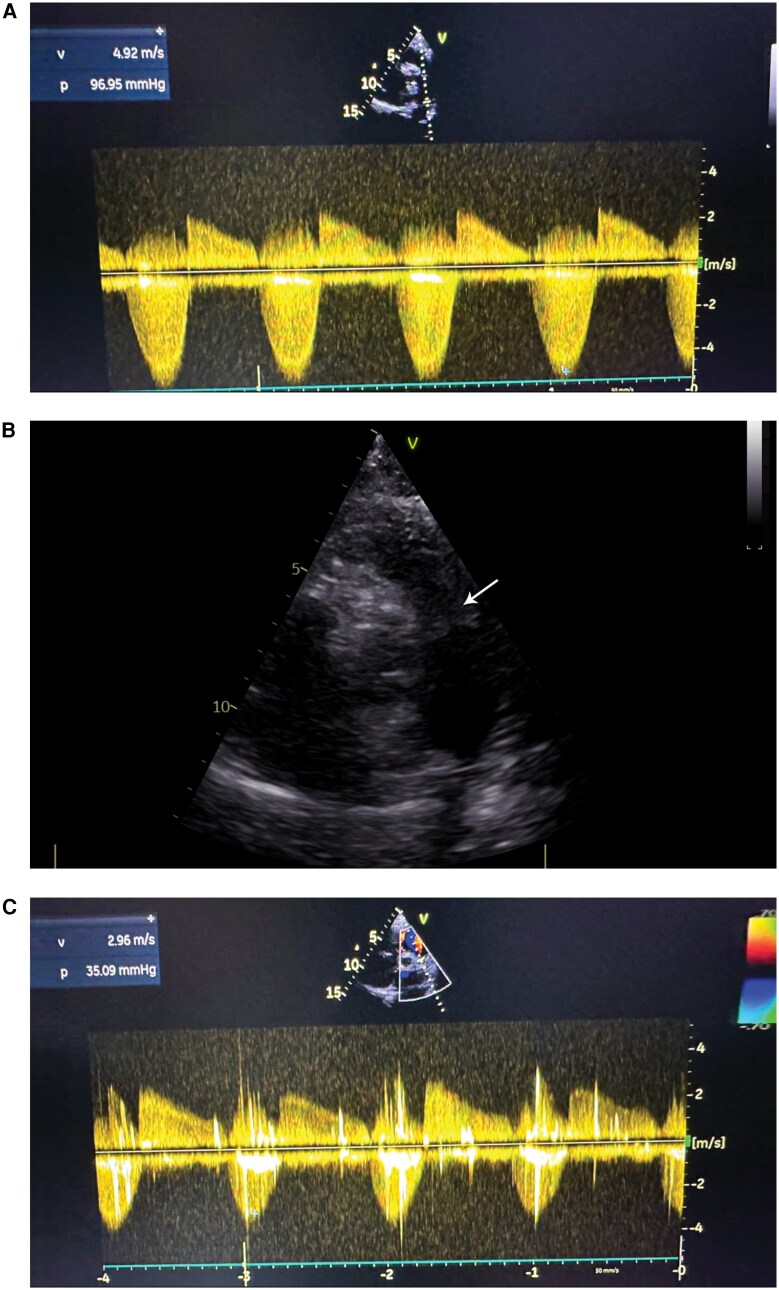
Transthoracic echocardiography of the pulmonary valve before and after balloon pulmonary valvuloplasty. (*A*) Continuous-wave Doppler echocardiography across the pulmonary valve demonstrating severe pulmonary stenosis with a peak gradient of 97 mmHg prior to intervention. (*B*) Parasternal short-axis echocardiographic view of the pulmonary valve demonstrating restricted systolic opening with commissural fusion (arrow). See Supplementary material online, *Video*  S1 for dynamic visualization. (*C*) Continuous-wave Doppler echocardiography across the pulmonary valve following balloon pulmonary valvuloplasty showing a marked reduction in the peak gradient to 35 mmHg.
